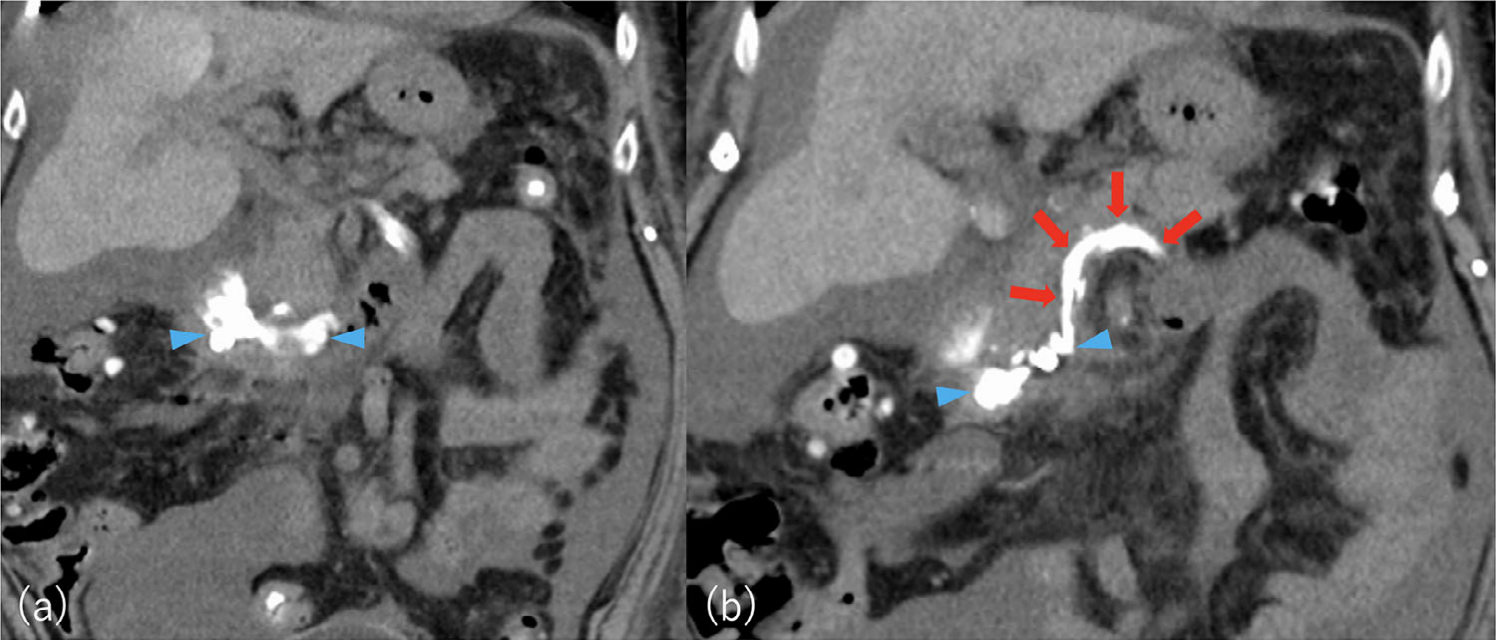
Computed tomography (CT) image after endoscopic ultrasonography (EUS)‐guided variceal therapy. (a) *N*‐butyl cyanoacrylate (NBCA) distributed within duodenal varices. (b) NBCA distributed within the duodenal varices and feeder vein originating from the distal superior mesenteric vein (SMV), the SMV, and the splenic vein. Blue arrowhead: NBCA distributed within the duodenal varices. Red arrow: NBCA distributed within the feeder vein (originating from the distal SMV), the SMV, and the splenic vein.
